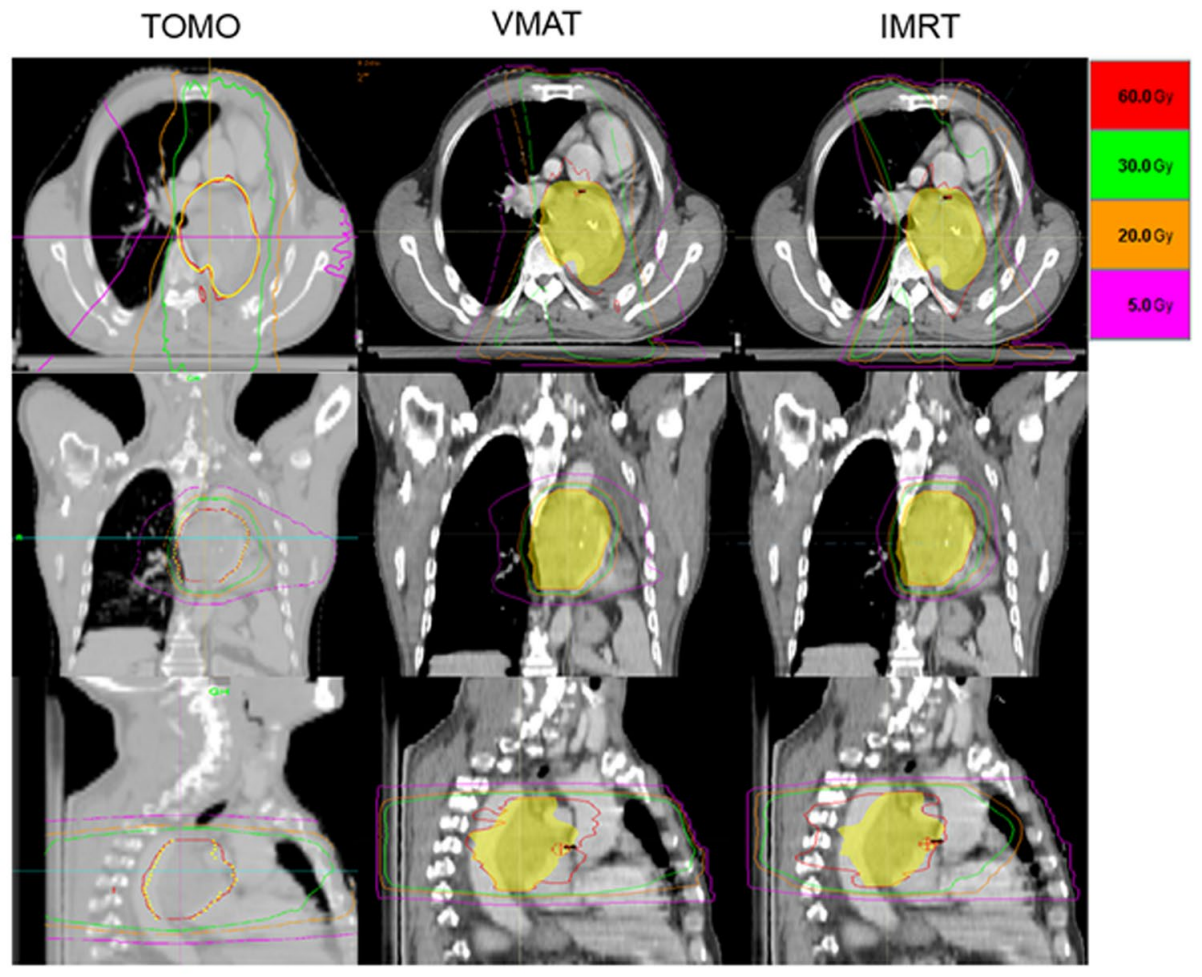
Typical isodose distributions for helical tomotherapy (TOMO), volumetric-modulated arc therapy (VMAT), and intensity-modulated radiotherapy (IMRT) plans for a patient showing the same CT slice. The planning target volume was painted in yellow. The pink, orange, green and red lines represent the dose curves of 5, 20, 30, and 60 (the prescription dose) Gy, respectively.
